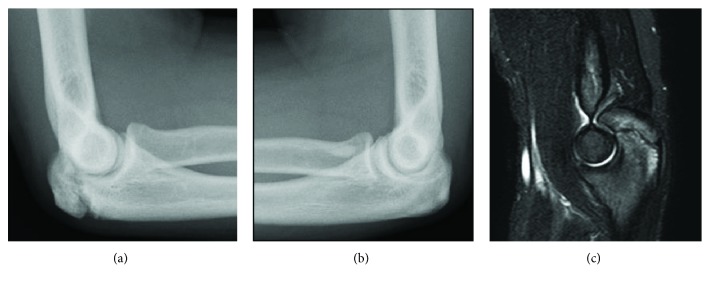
Radiographs of the elbows of a 15-year-old male patient (Patient 2). (a) Lateral radiograph of the right elbow showing delayed union of the olecranon apophysis. (b) Comparison view of the left elbow showing a completely fused apophysis. (c) Sagittal plane magnetic resonance imaging scan (short TI inversion recovery: STIR).
